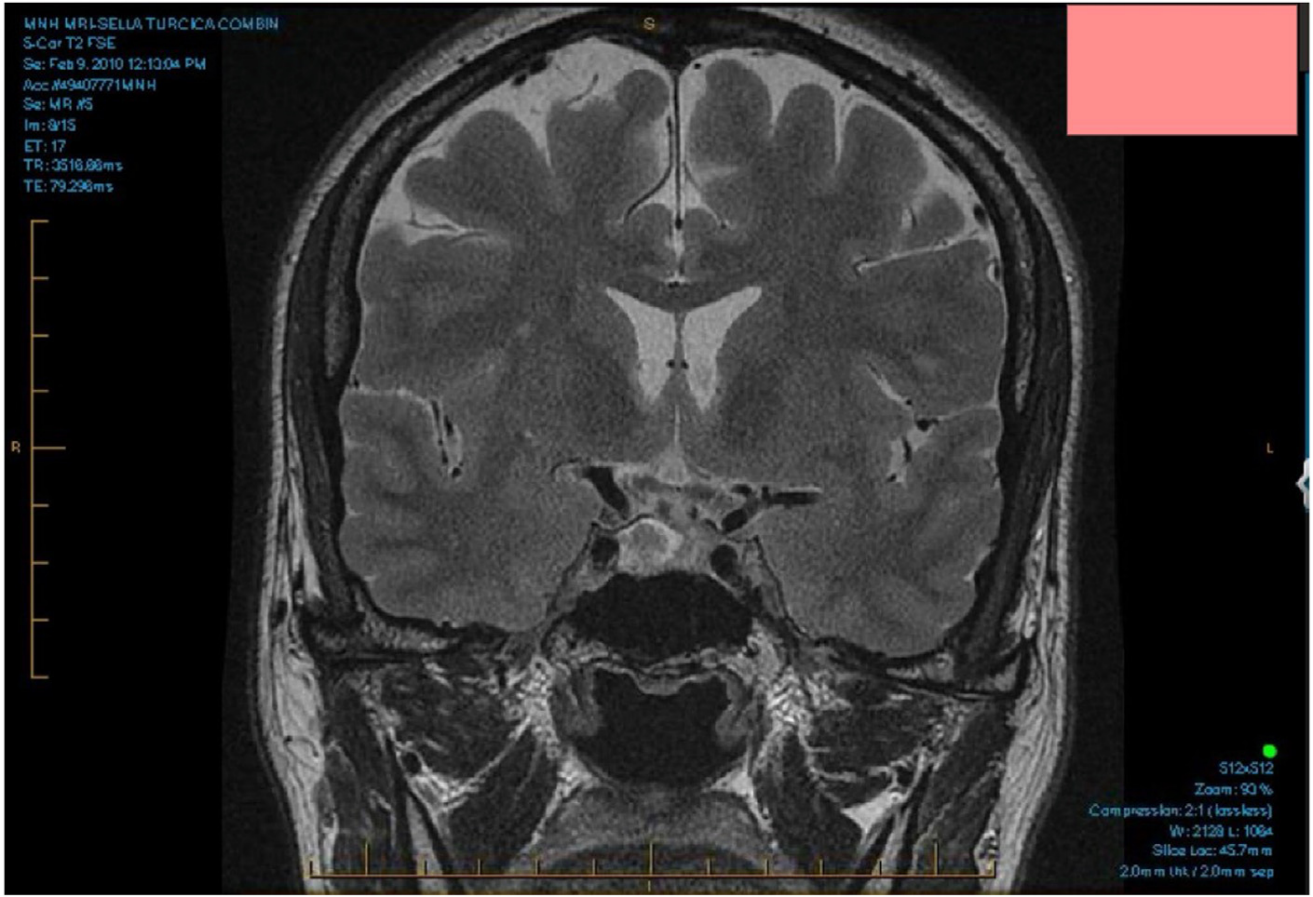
Preoperative brain magnetic resonance imaging (MRI); Showing a 1.8 cm pituitary adenoma in right hemianterior pituitary gland.
